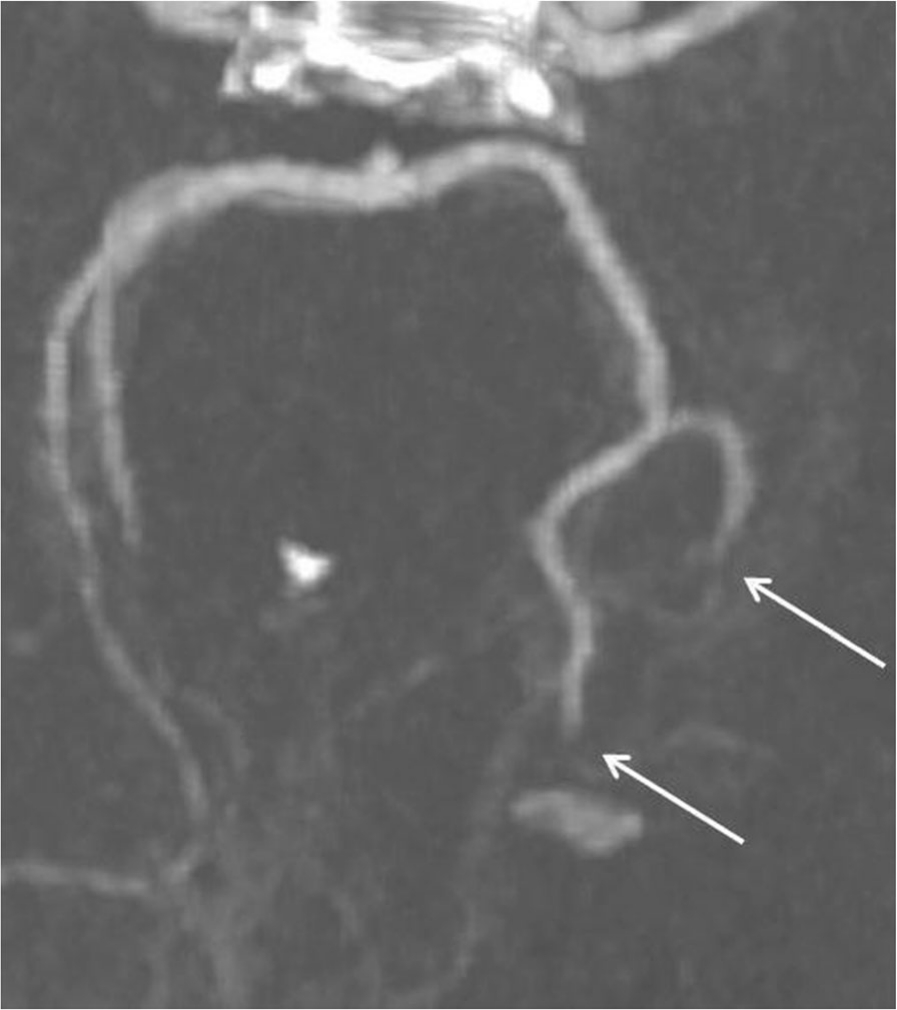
CTA showing occlusions of the P2 and P3 segments of the left posterior cerebral artery
